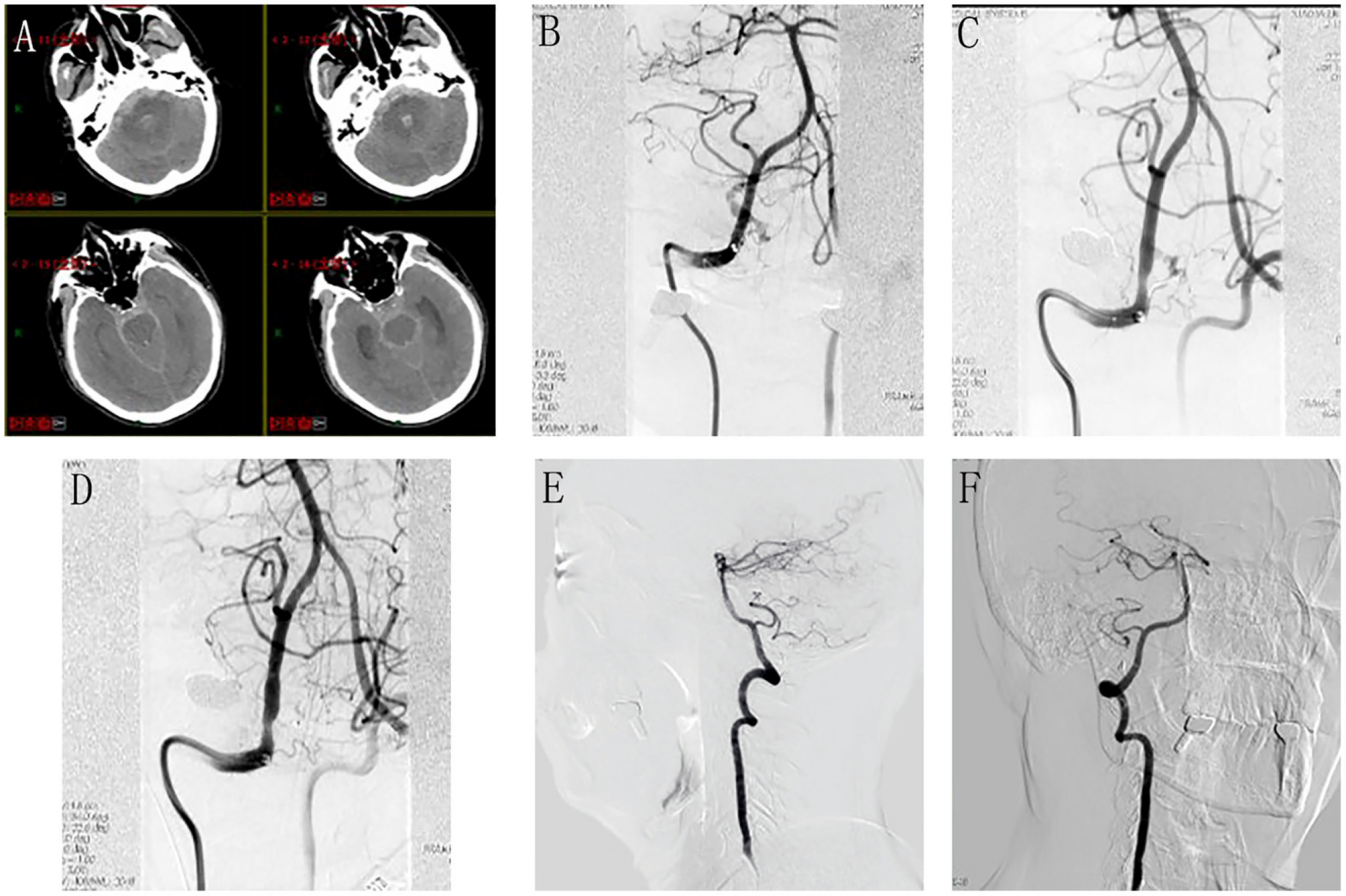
Perioperative neuroimages of Case 13. Emergent cranial CT revealed diffuse SAH and 4th ventricular hematoma (A). Emergent cerebral DSA revealed a DAVF located at the foramen magnum supplied by the meningeal branch of the right VA, draining into the enlarged tortuous medullary vein (B). During the procedure, the microcatheter tip was advanced to reach the optimal position (C); the injection of Onyx was continued until the DAVF was completely obliterated (D). Follow-up angiography at 6 months did not reveal any fistula residual or recurrence (E), lateral view; (F), anteroposterior view.
